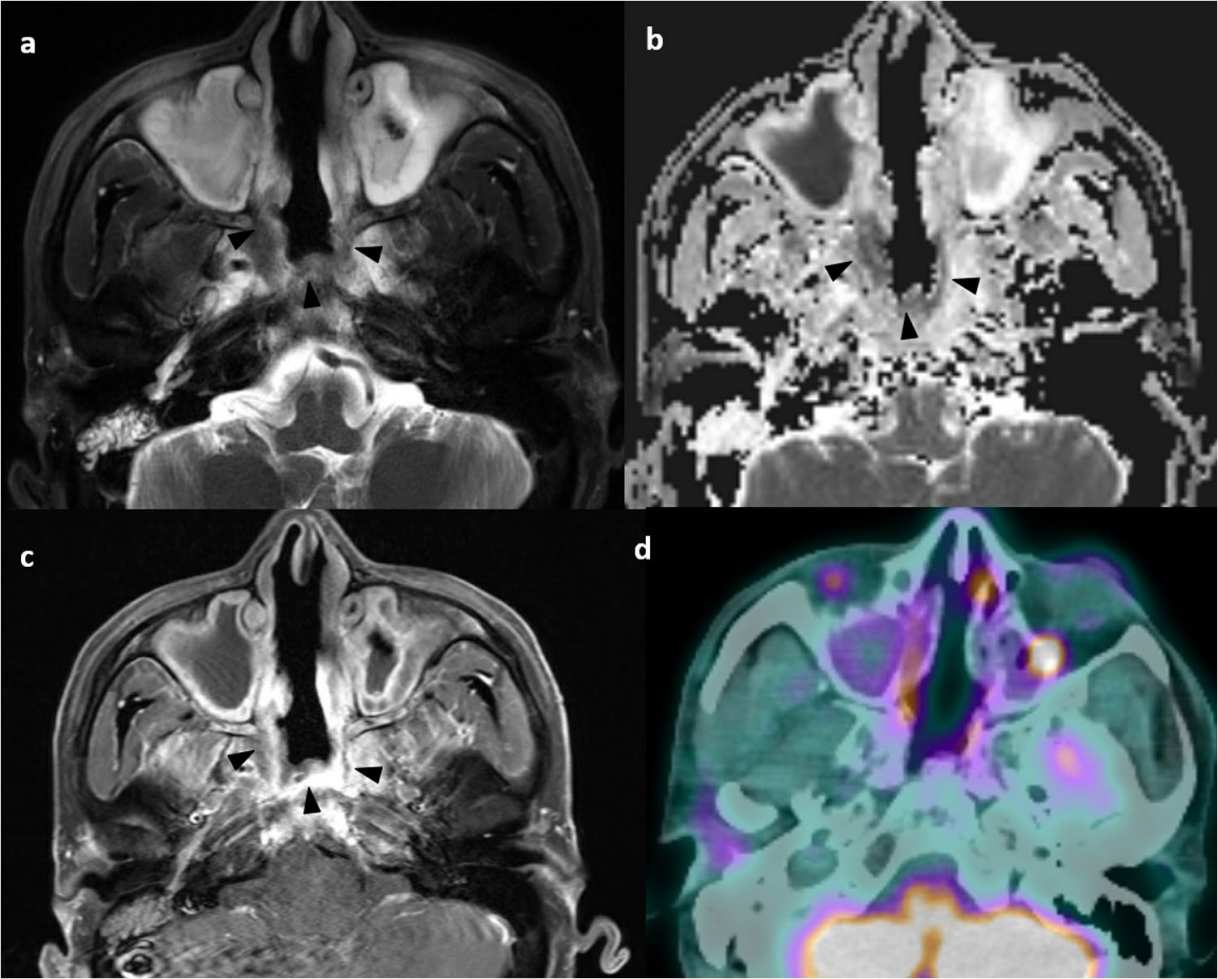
Same patient as Fig. 16. Recurrent tumor after endoscopic nasopharyngectomy in a 51-year-old man who had NPC (clinical T3N2M0) and completed chemoradiation in 2022. He had recurrent NPC (rT1N0M0) one-year after completion of treatment and underwent transnasal endoscopic nasopharyngectomy. A surveillance MRI was performed at 18-month post-surgery. Axial T2-weighted image with fat suppression at the superior resection margin shows infiltrative intermediate signal involving bilateral posterior and lateral walls of the nasopharynx (arrowheads). There is also perifocal patchy hyperintense signal involving the bilateral pterygoid bases and the clivus (a). ADC map shows the infiltrative soft tissue along bilateral nasopharyngeal walls to be hypointense (arrowheads) with hyperintensity on the high b-value DWI (not shown), in keeping with restricted diffusion (b). Axial post-contrast T1-weighted image with fat suppression shows the moderate enhancement of the soft tissue along bilateral nasopharyngeal walls (arrowheads), surrounded by marked enhancement in the adjacent soft tissue and the bone (c). Findings are suggestive of recurrent tumor along the nasopharyngeal walls on the background of post-operative change. Axial fusion FDG-PET/CT confirms the presence of hypermetabolic tumor along the nasopharyngeal walls. Otherwise no nodal or distant metastasis (d). Biopsy confirmed recurrent undifferentiated carcinoma
